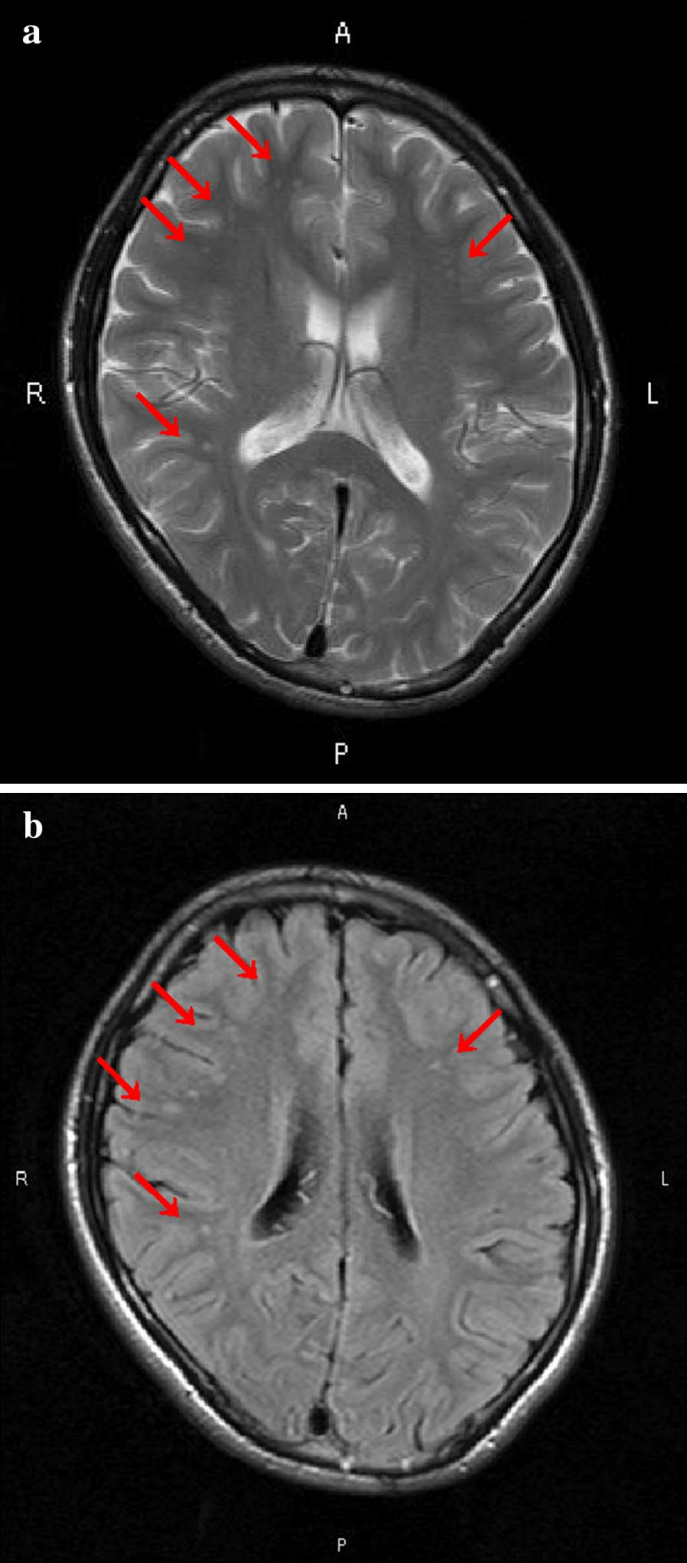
Magnetic resonance imaging (MRI) of the brain: a T2-weighted image. b fluid-attenuated inversion recovery (FLAIR) image
